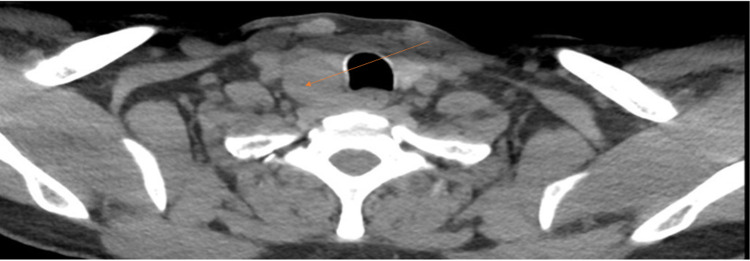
Chest CT on Day 5 of Admission Impression: Large predominantly cystic lesion with tiny enhancing internal nodular components lying on the external aspect of the inferior right lobe of the thyroid most likely representing a cystic parathyroid adenoma
